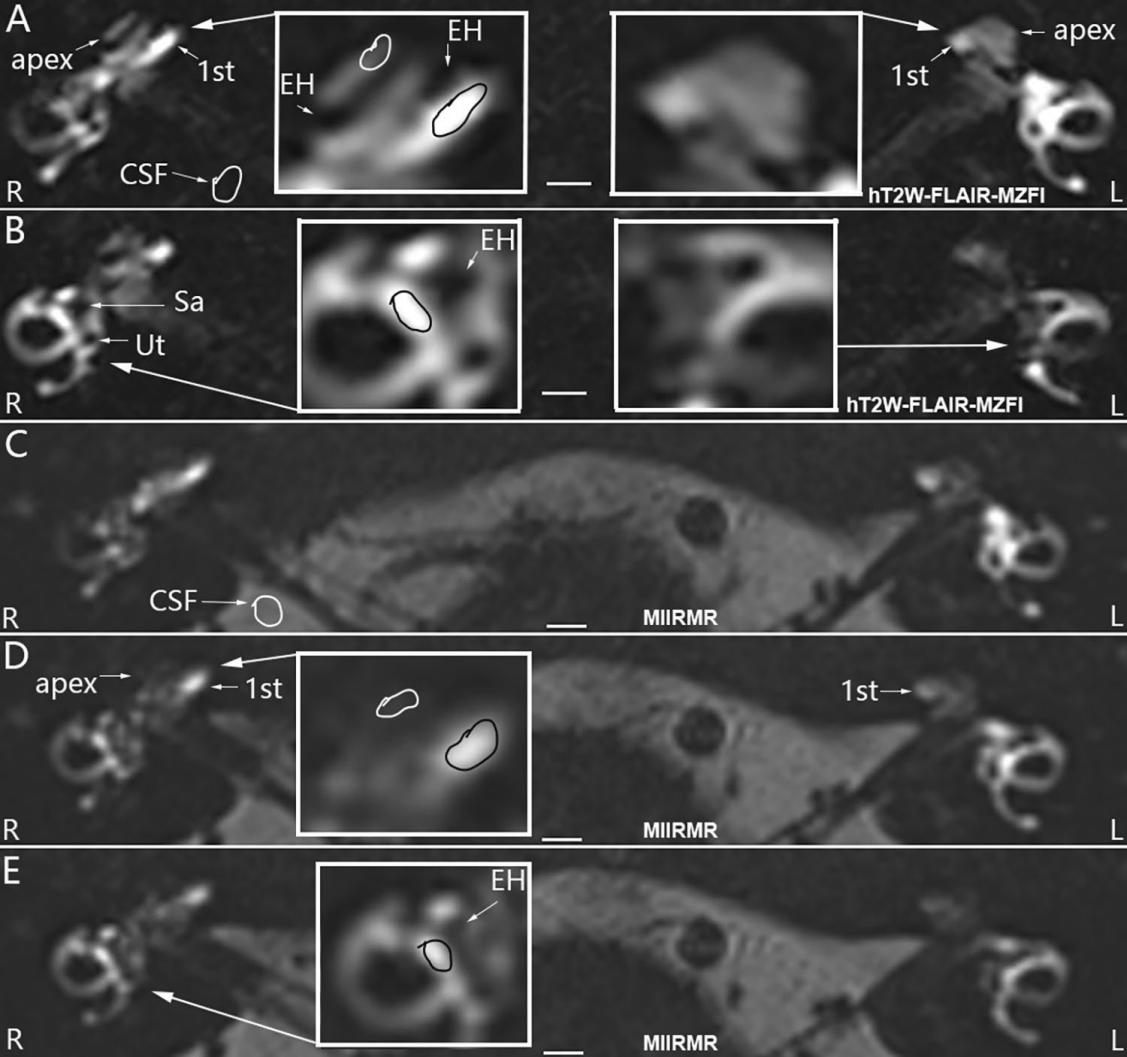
Comparison of hT_2_W–FLAIR–MZFI and MIIRMR 24 h after targeted delivery of 20-fold-diluted Gd–DTPA in a patient with Meniere’s disease. The patient suffered from episodic vertigo for 20 years and fluctuating right ear hearing loss for 1 year. The image quality and enhancement in the cochlear apex were significantly higher on MRI obtained with hT_2_W–FLAIR–MZFI A, B than on MRI obtained with MIIRMR C–E. The images acquired using MIRMR were obviously inhomogeneous C–E. Grade II cochlear endolymphatic hydrops (EH) was detected on the right (R) (zoom in A) using hT_2_W–FLAIR–MZFI but not with MIIRMR (zoom in D). Grade I vestibular EH was demonstrated in the right ear using both hT_2_W–FLAIR–MZFI (zoomed in B) and MIIRMR (zoomed in E). EH was not demonstrated in the left ear (L) imaged with either hT_2_W–FLAIR–MZFI (zoomed in A, B) or MIIRMR. Signal intensities in the regions of interest (circled areas in A, B, D, and E) were evaluated on a single slide for statistical analysis. *1st* basal turn, *CSF* cerebrospinal fluid, *Sa* saccule, *Ut* utricle. Scale bars = 3.0 mm
